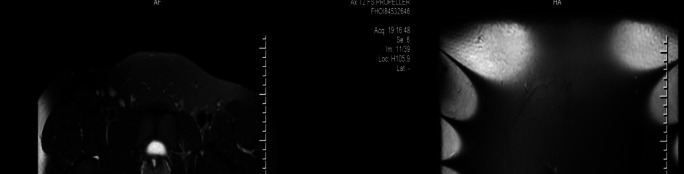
The follow-up MRI image, performed 3 months after laser therapy, showing the successful treatment outcome in a patient with recurrent pilonidal sinus previously operated using the Limberg flap technique
